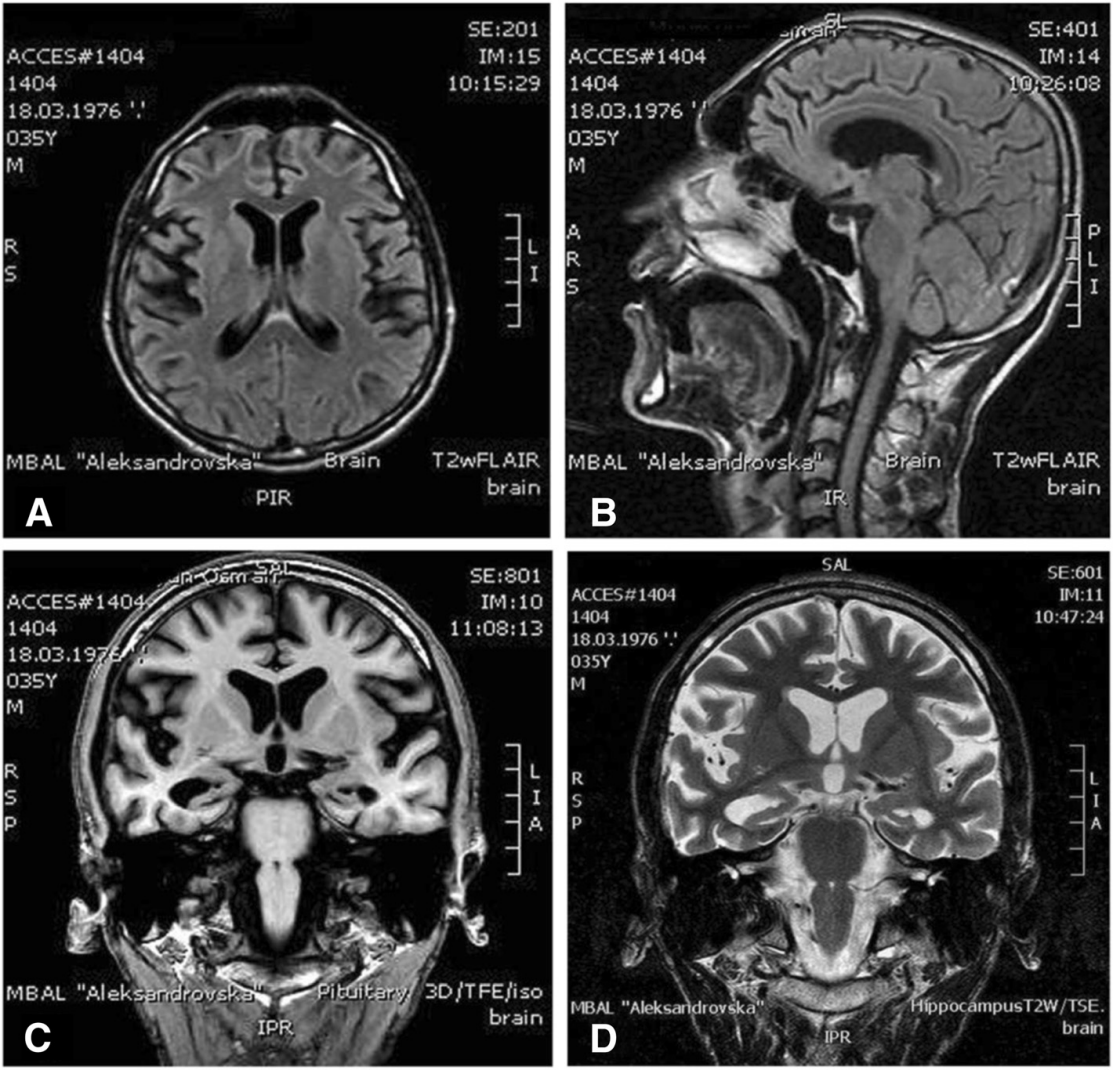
Magnetic Resonance Imaging (MRI) of the brain. A/ Axial fluid attenuated inversion recovery (FLAIR). B/ Sagittal fluid attenuated inversion recovery (FLAIR). C/ Coronal 3D -T1-TFE. D/Coronal T2WI – TSE. All images are showing marked diffuse loss of brain parenchyma including mesiotemporal atrophy. Note that there are no areas of increased signal intensity in the FLAIR and T2W images.
